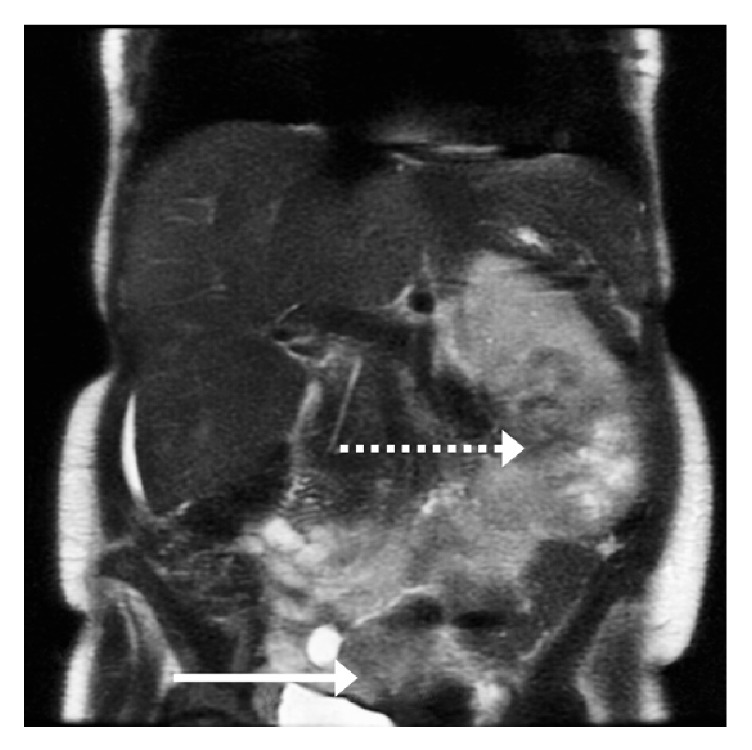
Axial & coronal view, CT of abdomen without contrast demonstrating multifocal left renal malignancy (dashed arrows) with metastasis to vagina as well as left hepatic lobe (solid arrows).
